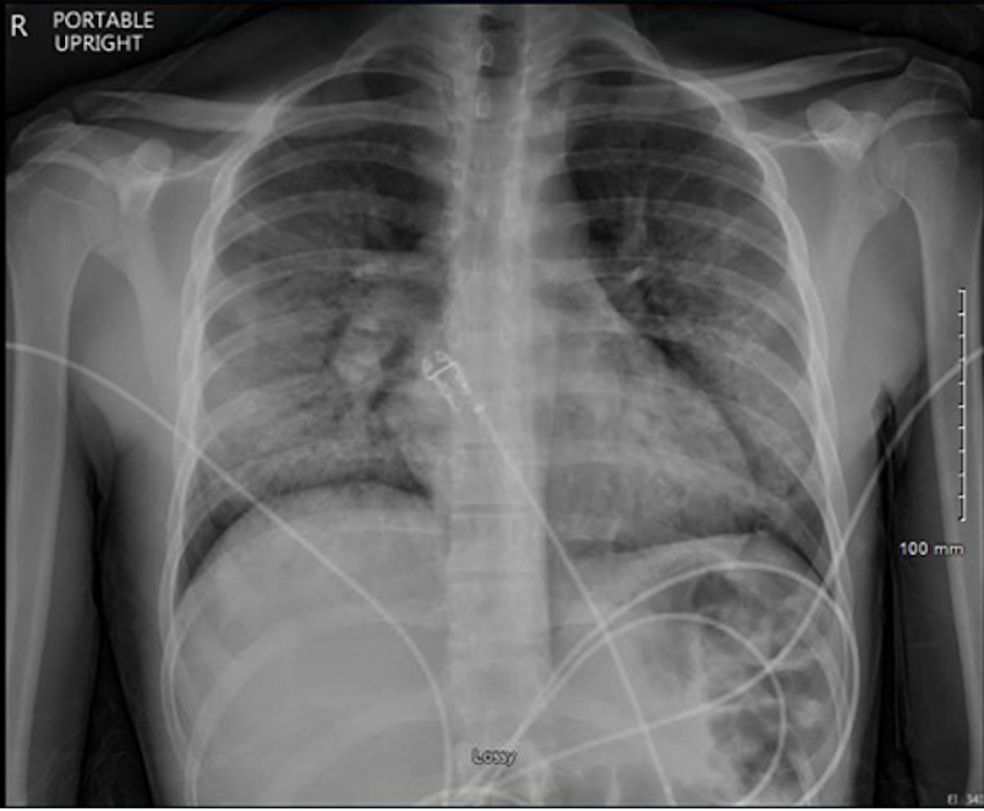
Chest X-ray at the time of admission showing bilateral patchy infiltrates.
